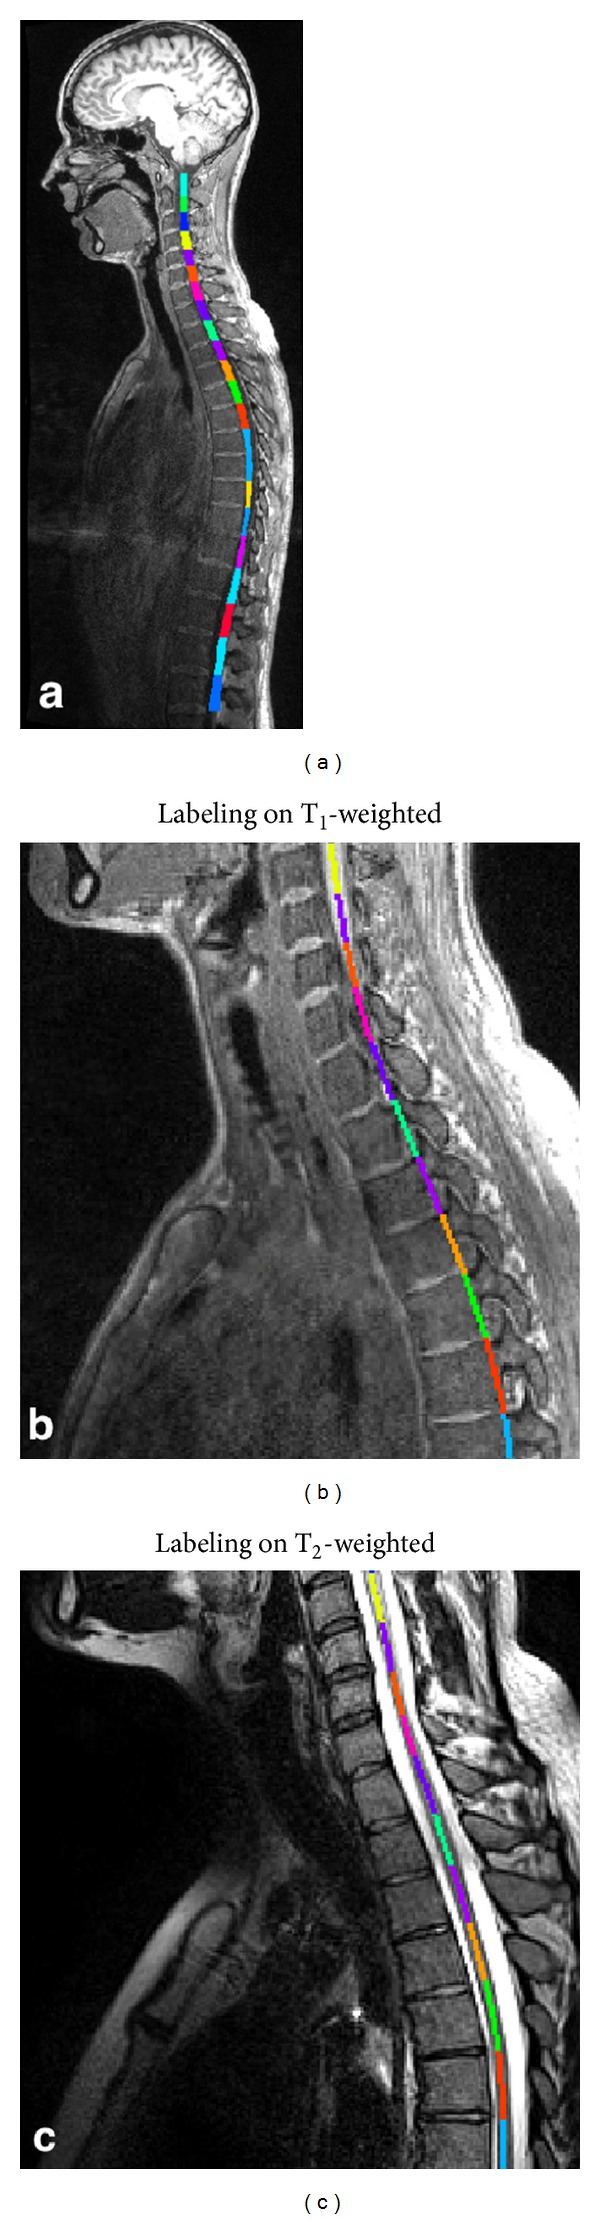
The left panel (a) shows T_1_-weighted MRI with an overlay of the labeled segmented spinal cord surface between C2 and L2. A zoomed panel shows the labeled centerline between C4 and T2 vertebrae for the T_1_-weighted (b) and the T_2_-weighted images (c).
